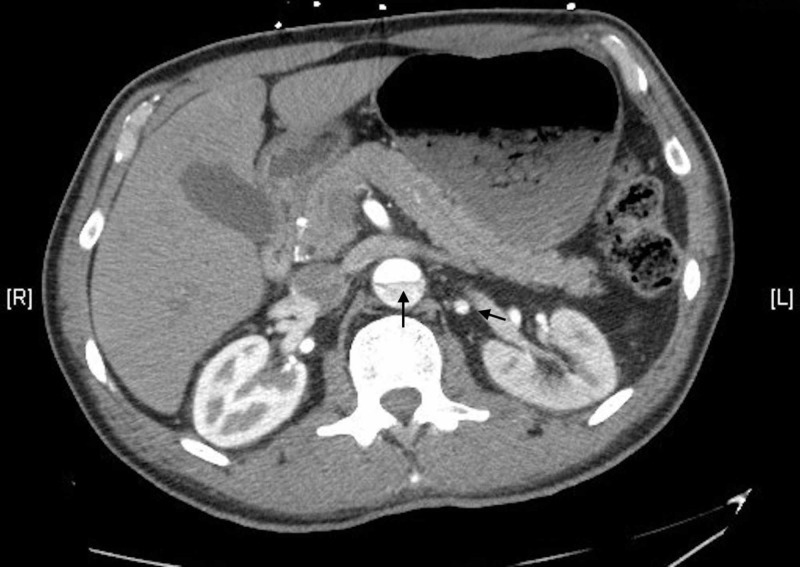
CT image of dissection extending to distal abdominal aorta and iliac artery CT, computed tomography
